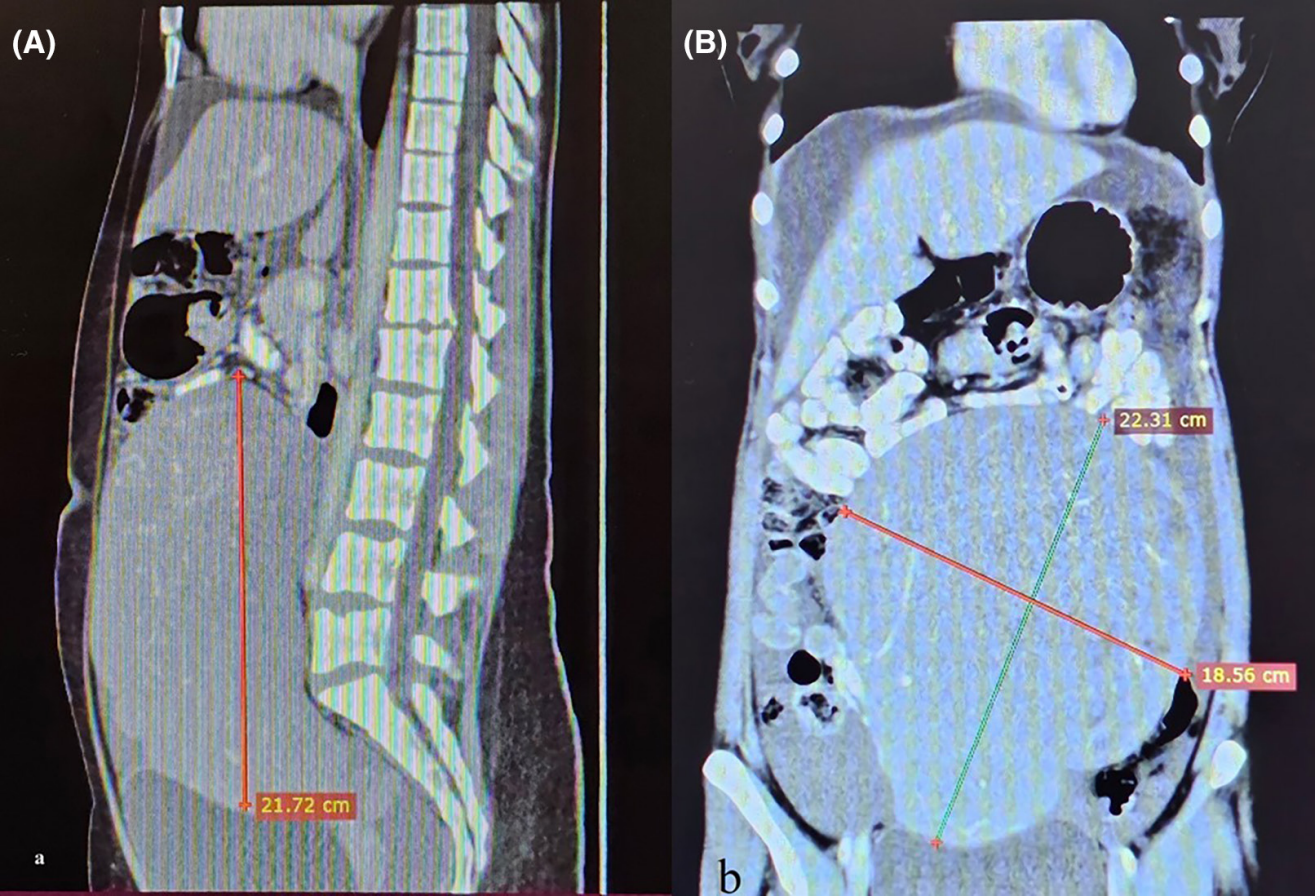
(A) Sagittal and (B) coronal computed tomography images showing intra‐abdominal mass measuring 22 cm (red arrow). Note the upward placement of other intra‐abdominal organs.
